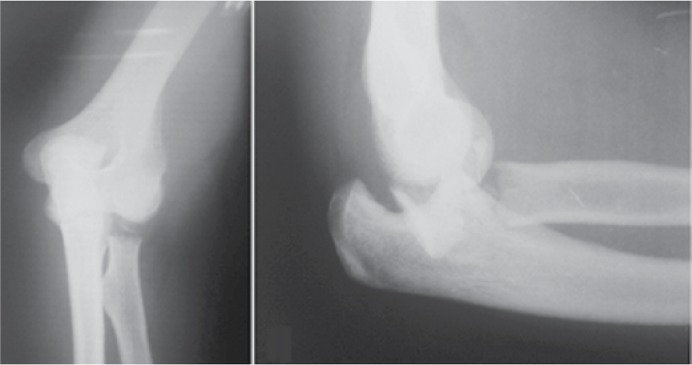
Profile and AP X-Ray showing the terrible triad of the elbow.
